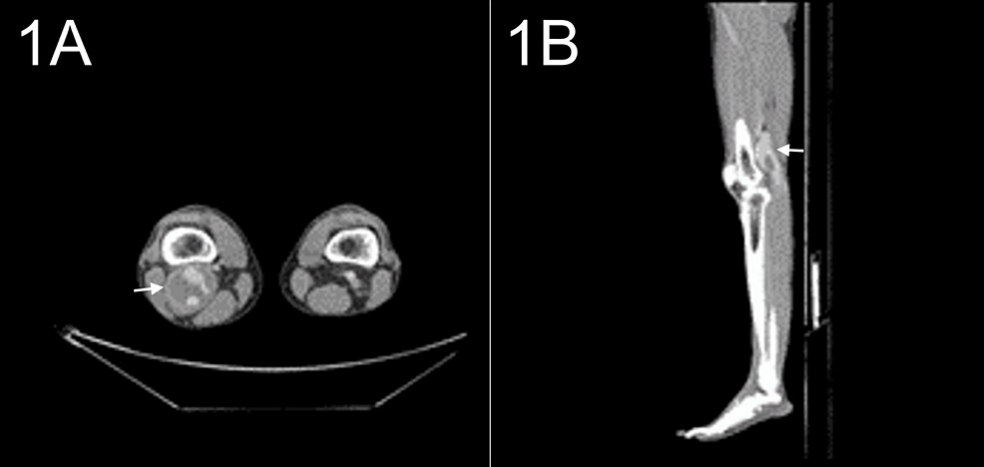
CT angiogram of lower extremities CT angiogram of the patient's lower extremities identified a 5x5 cm popliteal artery aneurysm (1A) and distal thrombosis, leaving inadequate outflow into the tibioperoneal trunk (1B).
